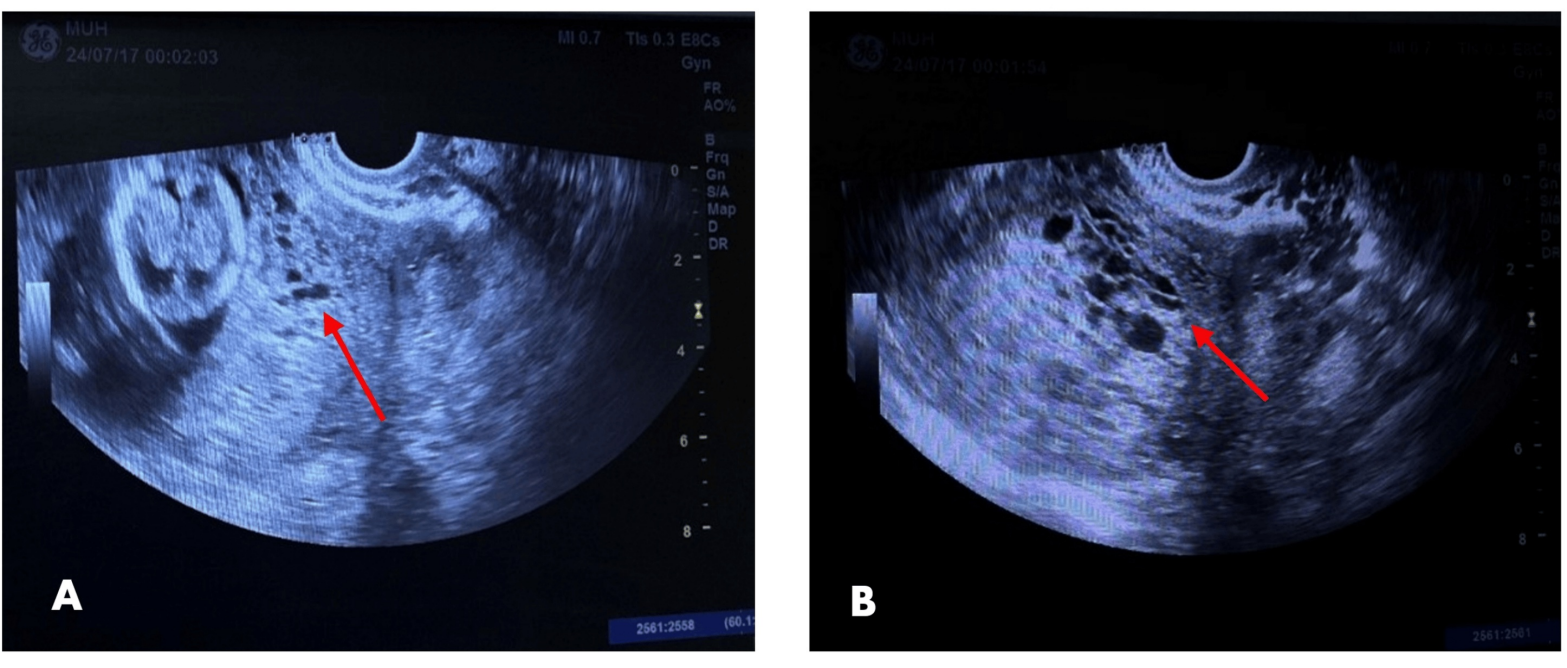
Ultrasound scan at 12 weeks of gestation (A) Ultrasound image showing a grape-like appearance of a coexisting fetus (suspected to be a hydatidiform mole) located at the uterine fundus (arrow). (B) Sonographic image showing placenta with multiple cystic spaces (arrow).
